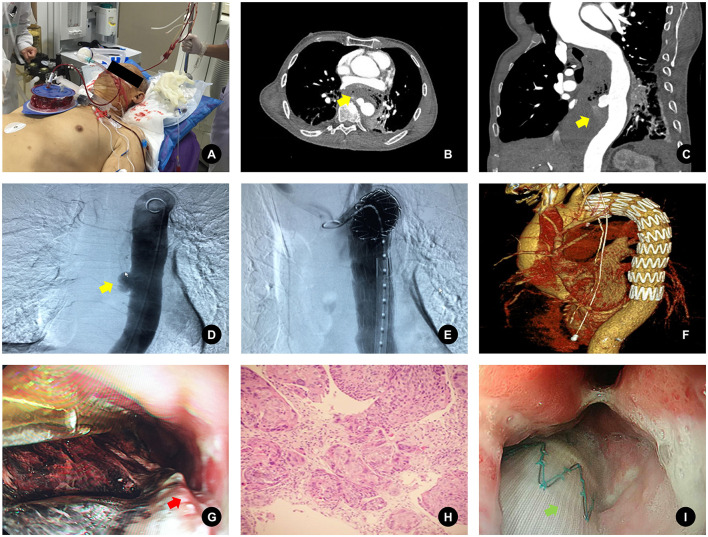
Main diagnostic and therapeutic processes of the case. (A) The patient had massive hematemesis on emergency examination. (B) Chest axial enhanced CT showed thoracic aortic aneurysm and contrast medium extravasation (yellow arrow). (C) Thoracoabdominal aorta CTA shows thoracic aortic aneurysm and contrast medium extravasation (yellow arrow). (D) DSA showed thoracic aortic aneurysm-like and contrast extravasation (yellow arrow). (E) The DSA guidewire was successfully placed. (F) Thoracic endovascular aortic repair (TEVAR) was performed using a COOK ZTEG-2PT-30-200 covered stent graft. (G) Gastroscopy on the second day after emergency intervention showed a giant ulcer covered with massive blood clots in the gastric tube and biopsy (red arrow). (H) The pathological results of biopsy at the edge of gastric mucosa ulcer under gastroscopy were squamous cell carcinoma. (I) Repeat gastroscopy on the 10th day after the intervention showed that the ulcer had been cleaned and the stent was visible inside.
